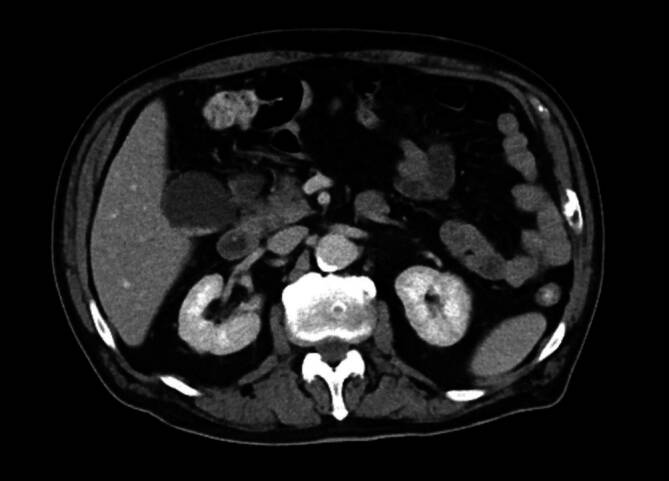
Computertomografie (CT) Abdomen mit Aerobilie und V.a. Vorliegen einer cholezystokolischen Fistel im Bereich des rechten Kolons
